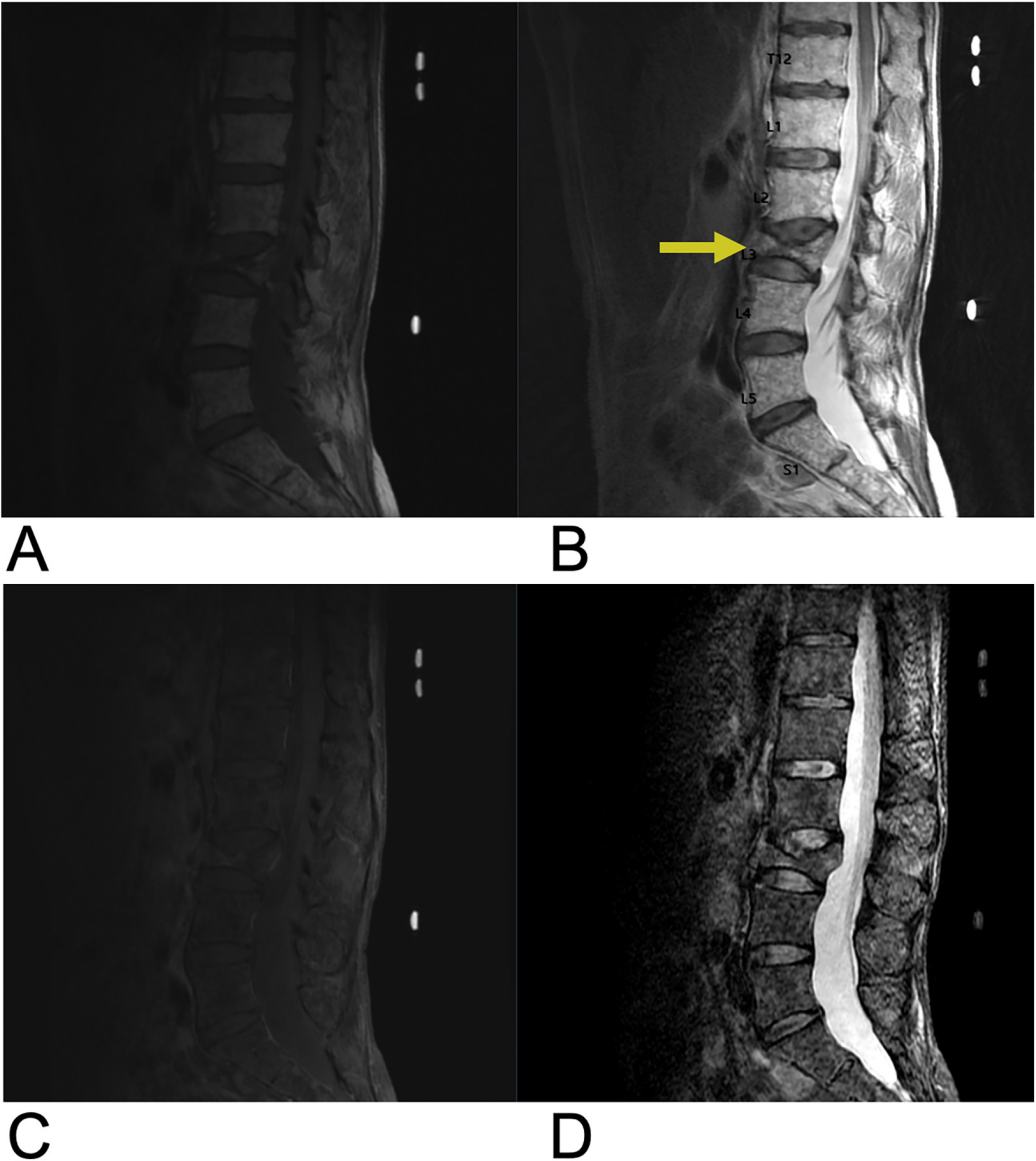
Multiple myeloma. Sagittal T1 (A), sagittal T2 (B), sagittal T1 + C (C), and sagittal STIR (D) MRI of the lumbar spine demonstrating diffuse T1 hypointense, heterogenous T2 hyperintense, and T1 + C enhancing lesions representing vertebral manifestations of multiple myeloma. There is a chronic L3 superior endplate pathologic fracture with approximately 30% loss of vertebral body height (yellow arrow) and retropulsion of posterior vertebral body cortex.
